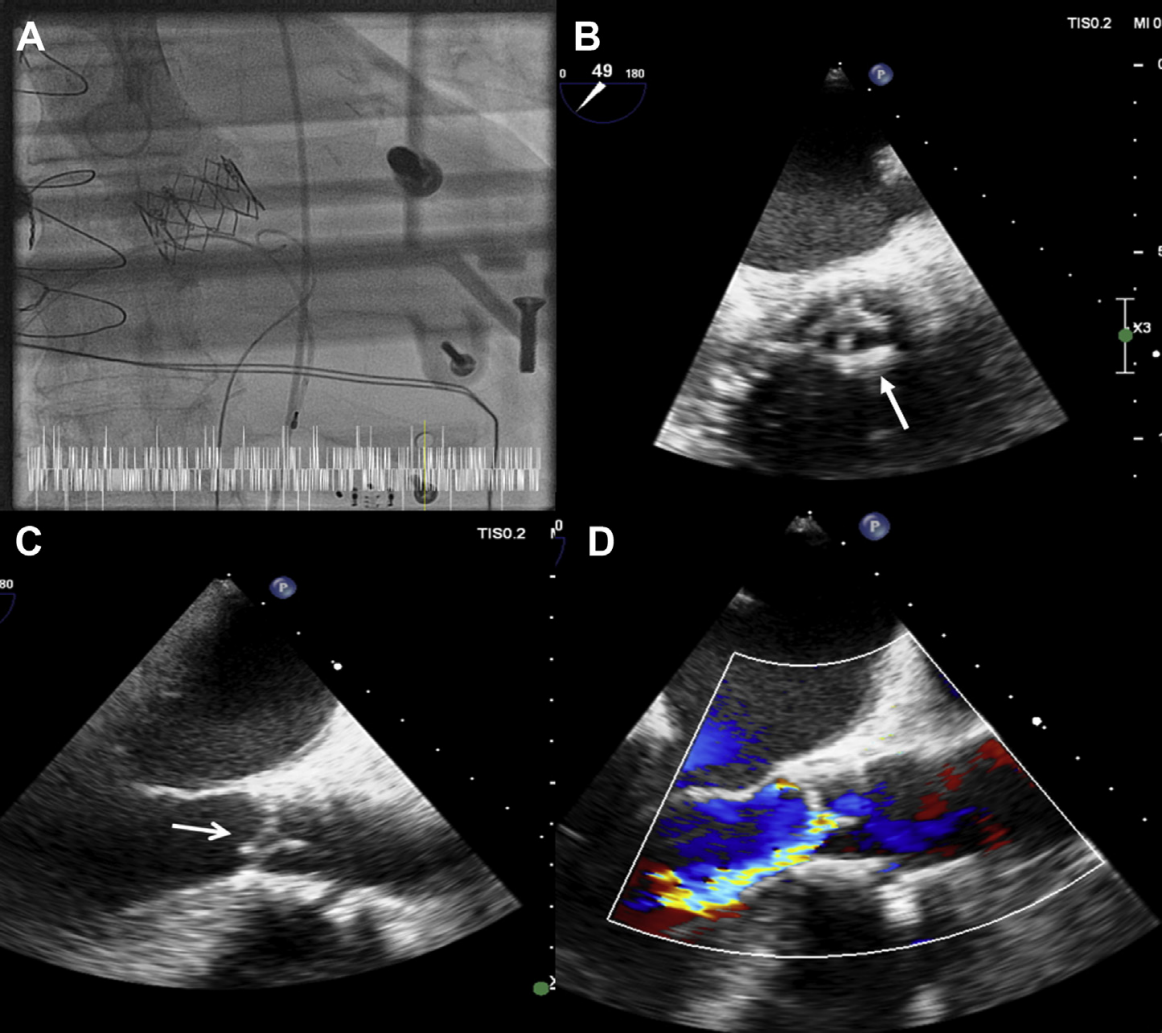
(A) Aortogram after deployment of 26-mm Sapien XT (Edwards Lifesciences Corp, Irvine, CA) valve in September 2012 for severe aortic stenosis showed absence of aortic regurgitation. (B) Transoesophageal echocardiogram (TEE) (March 2020), in the short axis view, showed good opening of the 26-mm Sapien XT valve but evidence of modest leaflet thickening, secondary to structural valve degeneration (arrow). (C) TEE (March 2020) in long axis view showed prolapse of a transcatheter heart valve cusp (arrow). (D) TEE (March 2020) in long axis view showed a jet of eccentric, severe aortic regurgitation (3D vena contracta area 0.31 cm^2^) due to cusp prolapse.
